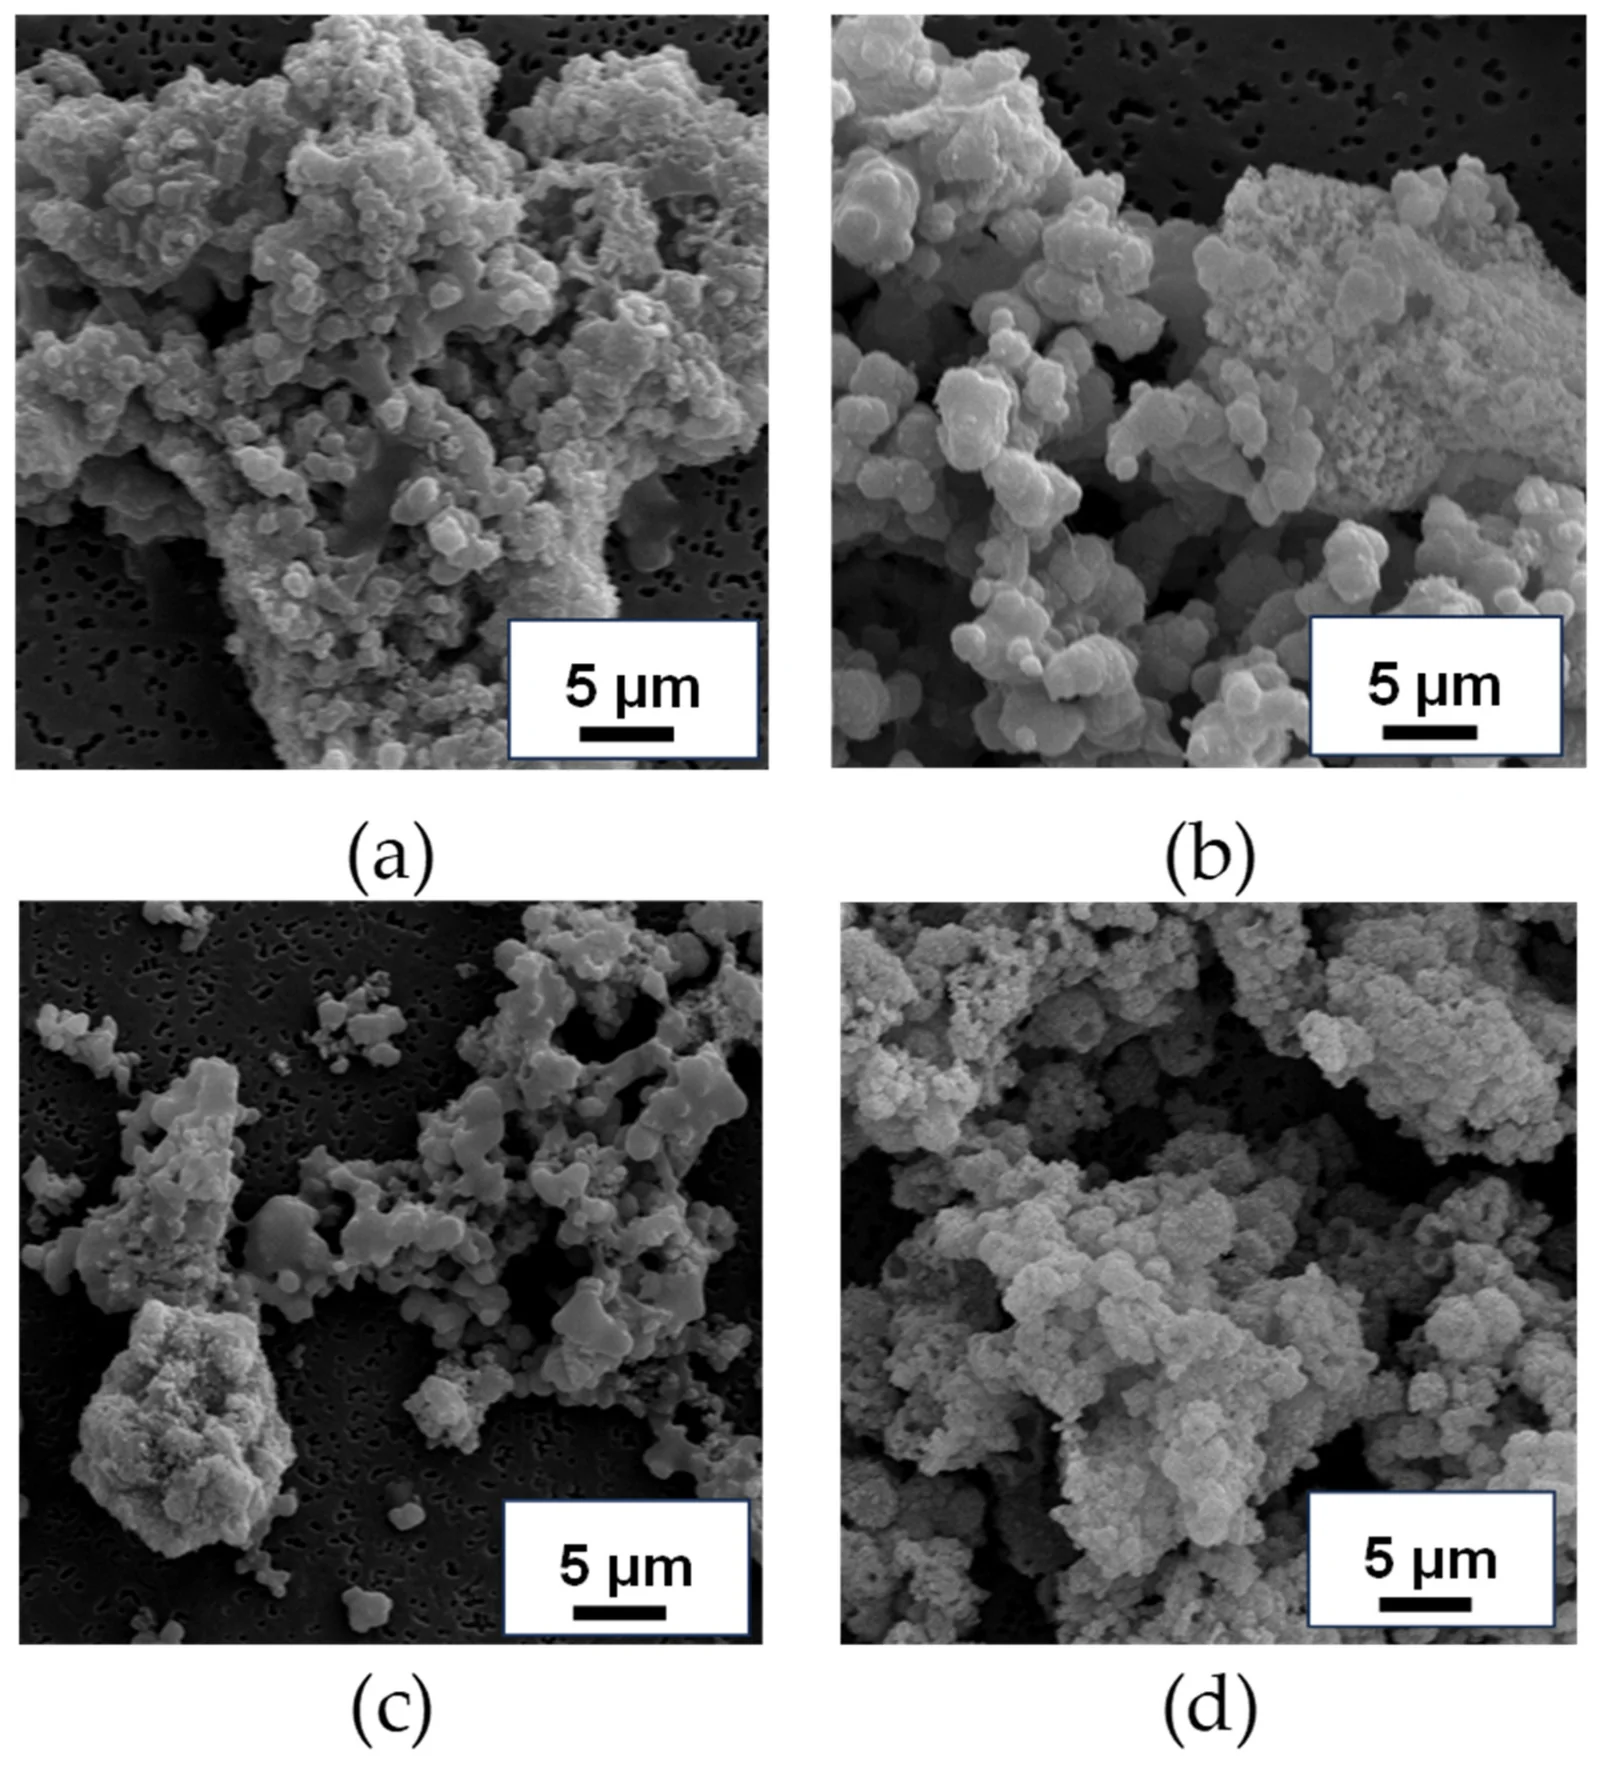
SEM images of calcium phosphates: (a)—AP, (b)—AP subjected to ultrasonication, (c)—AP-HS, and (d)—AP-HS subjected to ultrasonication.
